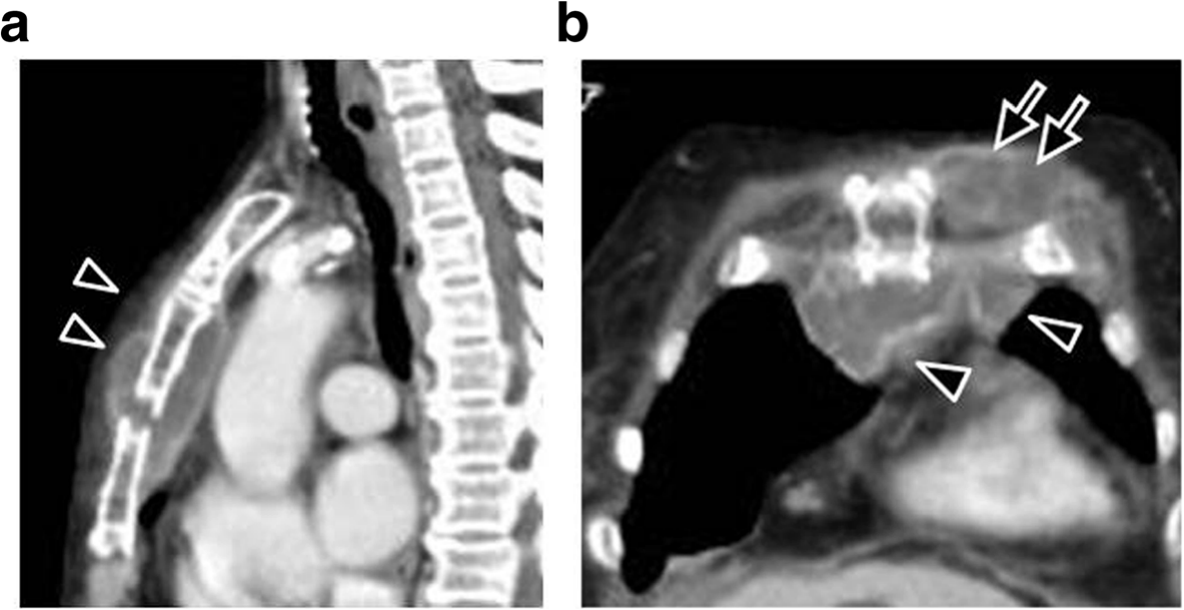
Contrast-enhanced computed tomography images 4 weeks after blunt chest trauma. a Sagittal image and b Axial image. The sternum was fractured and the mediastinal abscess can be seen anterior and posterior to the sternum (*arrowheads*) and extending to the left pectoralis muscles (*arrows*). Four weeks after traumatic accident
